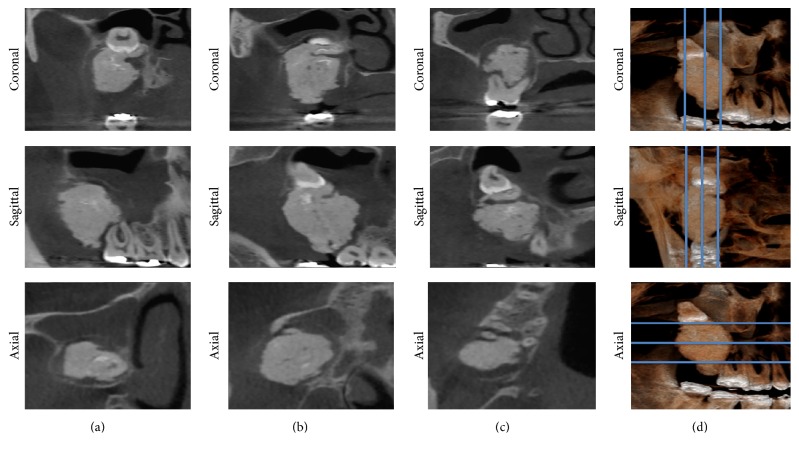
Multiplanar reconstruction of CBCT in three different regions from each view.
